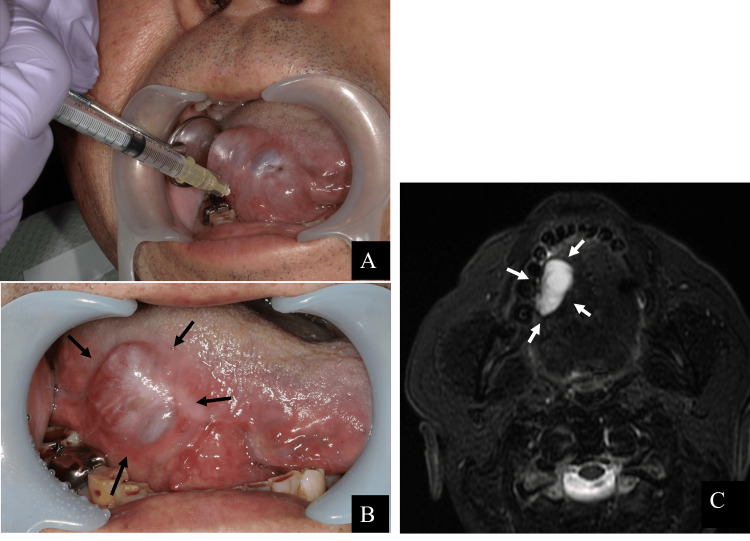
Treatment process of sclerotherapy with OK-432 for intraoral ranula. (A) An intraoral photograph was taken during initial OK-432 sclerotherapy, nine months after the initial visit (eight months after tongue cancer surgery). OK-432 1KE with 0.2 mL saline was injected into the ranula with a 30G needle. (B) Intraoral photograph was taken after the third dose of OK-432, 12 months after the initial visit (11 months after tongue cancer surgery), showing a recurrence of a 20 x 15 mm blue-purple mass. (C) Contrast-enhanced MRI, T2-weighted image was taken after the third dose of OK-432, 12 months after the initial visit. The mass had shrunk slightly but recurred.
